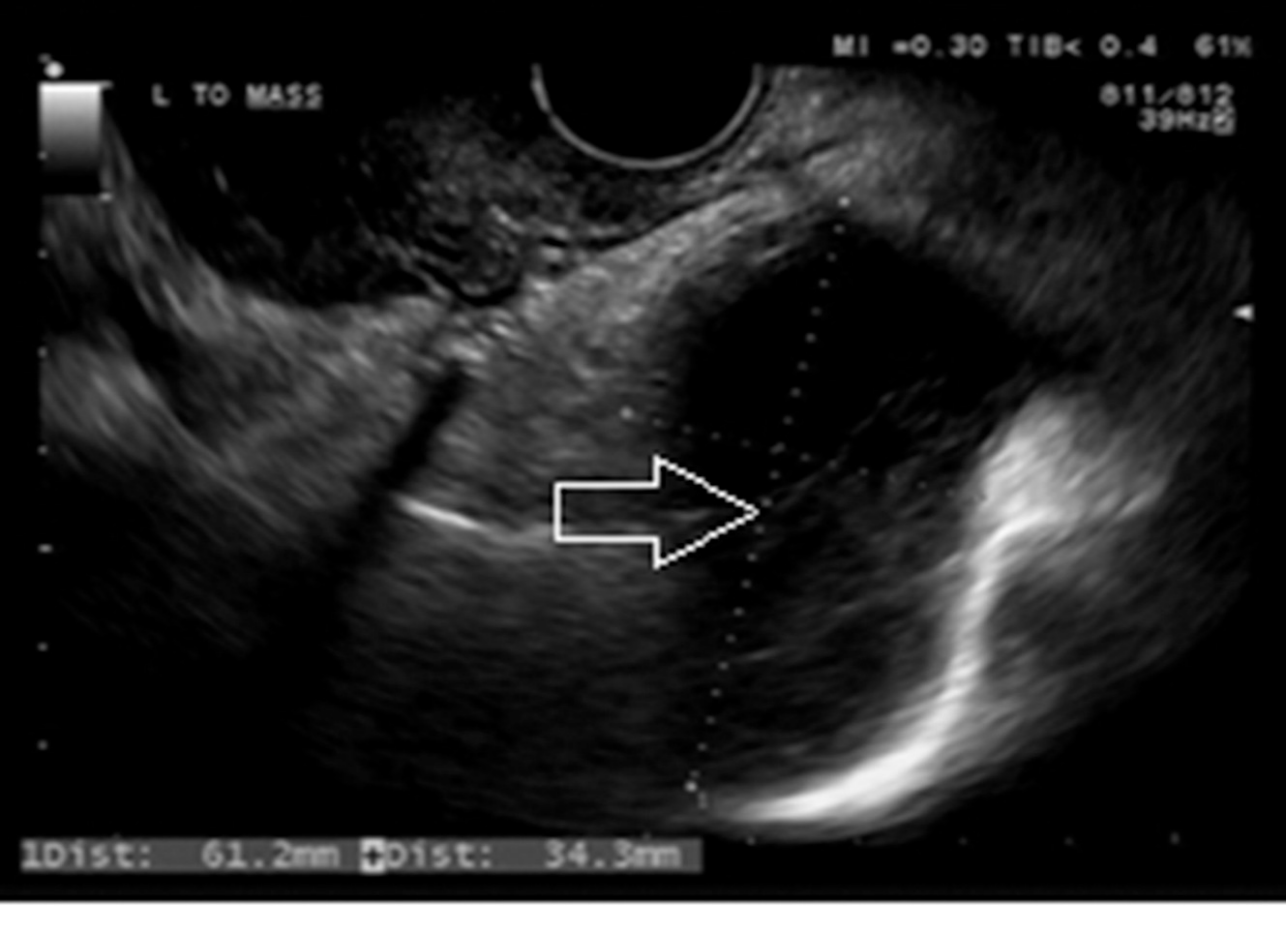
Transvaginal ultrasound scan showing the predominantly cystic left pelvic lesion (arrow) interpreted as a left-sided hydrosalpinx.
